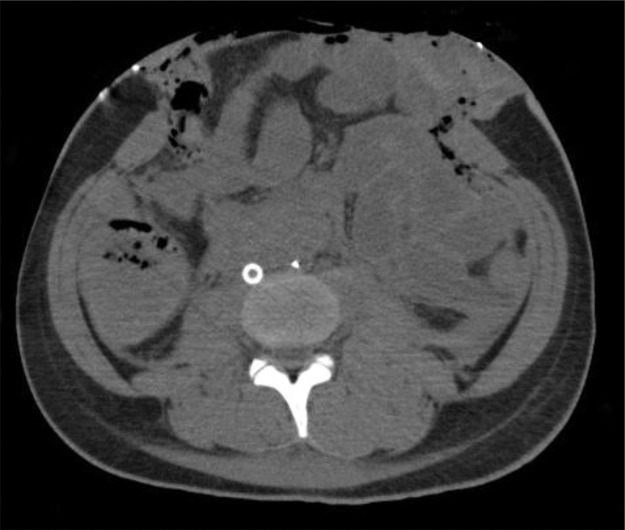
CT images after gauze packing and temporary closure using iodine drapes.
